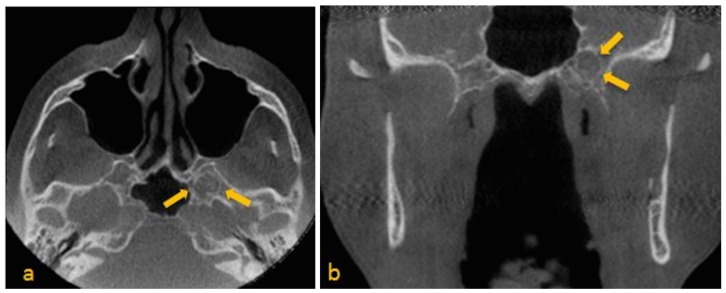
(a) Para-axial; (b) Paracoronal CBCT images show mixed density area in the left greater wing of the sphenoid adjacent to the sphenoid sinus. Note curvilinear calcifications within this area. (a) Para-axial view, shows suspected disruption of the vidian canal on the left side; (b) Paracoronal view, depicts vidian canal wider than the right side.
